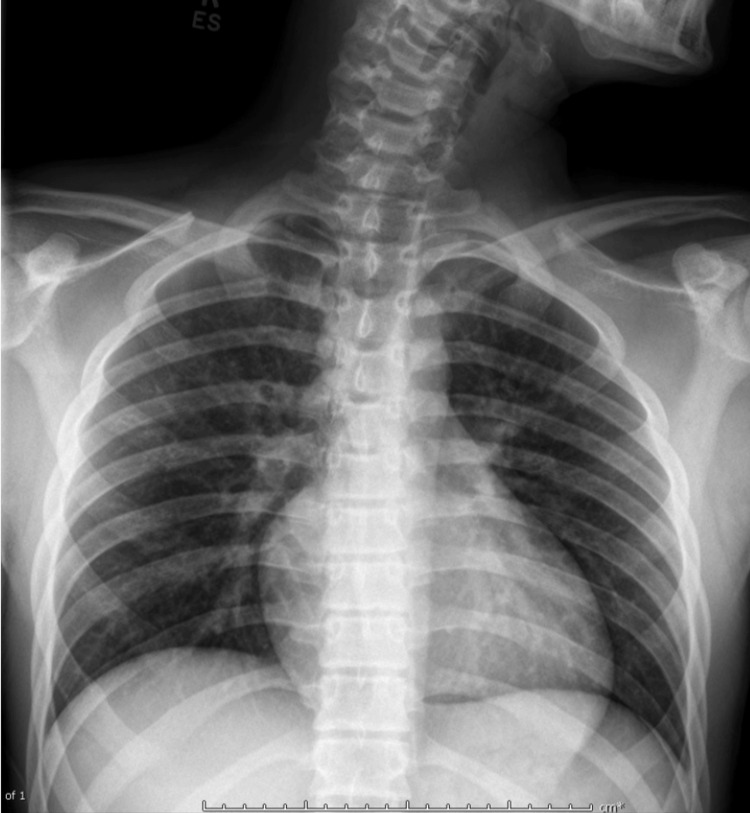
Initial plain, anteroposterior chest radiograph with negative findings.
